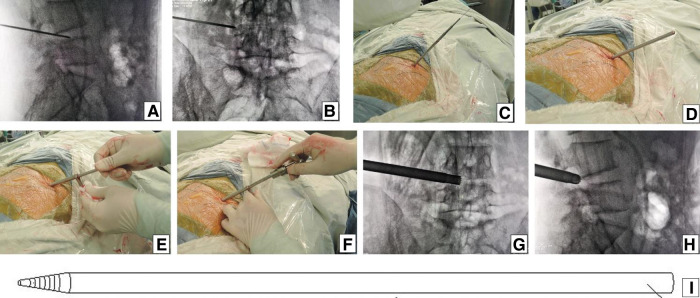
Guiding rod technique. After the puncture needle was inserted at 55° to the horizontal plane until the resistance disappeared, the C-arm view was taken to ensure that the tip of the puncture needle was in the posterior one-third of intervertebral space on (A) lateral x-ray and near the lateral border of the pedicle on (B) posteroanterior x-ray. (C) Over the guiding wire, stepwise-dilating cannulas were introduced to the anulus fibrosus beside the foramen. (D) Thick guiding rod of 6.3 mm diameter was inserted over the guiding wire and then adjusted into the foramen by moving the tip dorsally and cephalad after removing the guiding wire. (E) An 8.8-mm protective cannula was pushed over the rod to the facet joint area, docked at the facet, and pressed down. (F) A 7.5-mm reamer was introduced to remove the ventral bone of the articular process for enlarging the intervertebral foramen. When resistance disappears, the tip of reamer should exceed the medial border of the pedicle on (G) posteroanterior C-arm view and reach close to the posterior wall of the target disc on (H) lateral C-arm view. (I) Picture showing the stiff thick guiding rod of 6.3 mm diameter, which is easier to adjust the puncture trajectory than the soft puncture needle.
